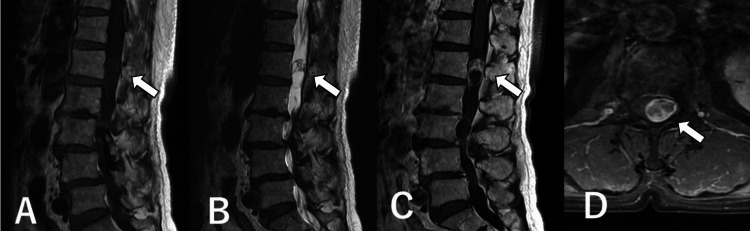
MRIs of lumbar tumor. A–C: Sagittal MRIs. An intradural extramedullary tumor (arrow) occupied the spinal canal and was low intense on the T1-weighted image (A) and high intense on the T2-weighted image (B). The contrast-enhanced T1-weighted image shows that a part of the tumor (arrow) consisted of cystic components, and the parenchymal component had a contrast effect (C). D: Axial fat-saturated contrast-enhanced T1-weighted image at L1. The tumor (arrow) compresses the spinal cord from the left side.
